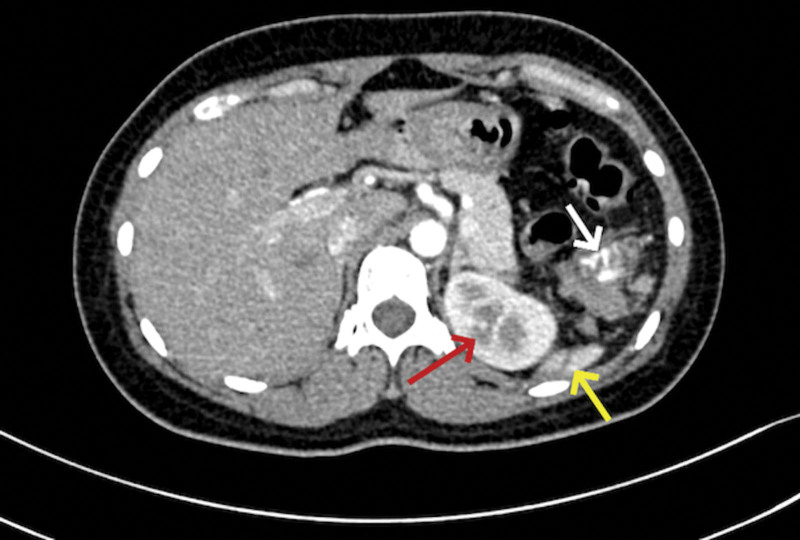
Contrast CT (arterial phase) showing the lesion at the same location measuring ~46 mm × 40 mm × 56 mm, it was surrounded by blood vessels (white arrow indicated the lesion; yellow arrow indicated the spleen; red arrow indicated the left kidney). CT = computerized tomography.
